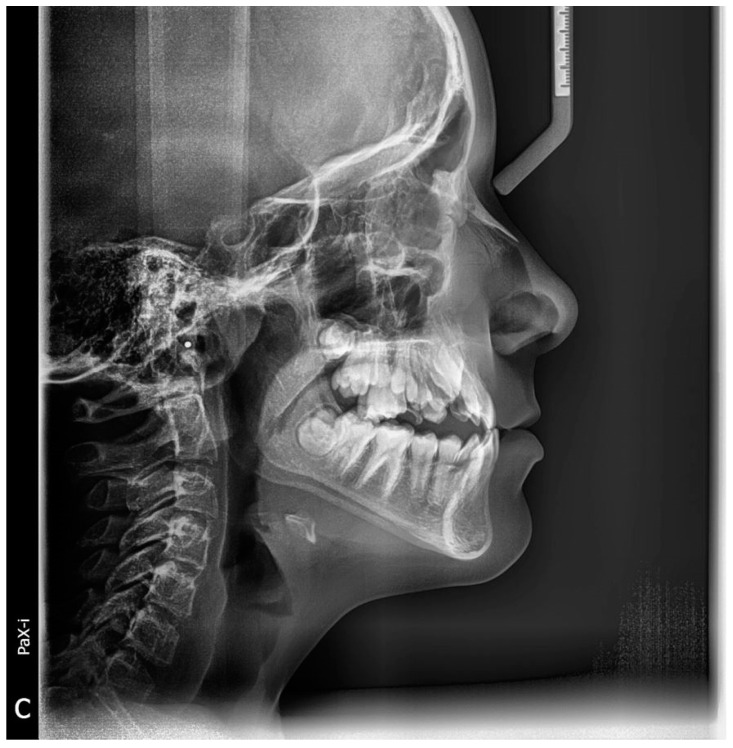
A cephalometric radiograph of the same patient revealed cervical vertebral maturation.
